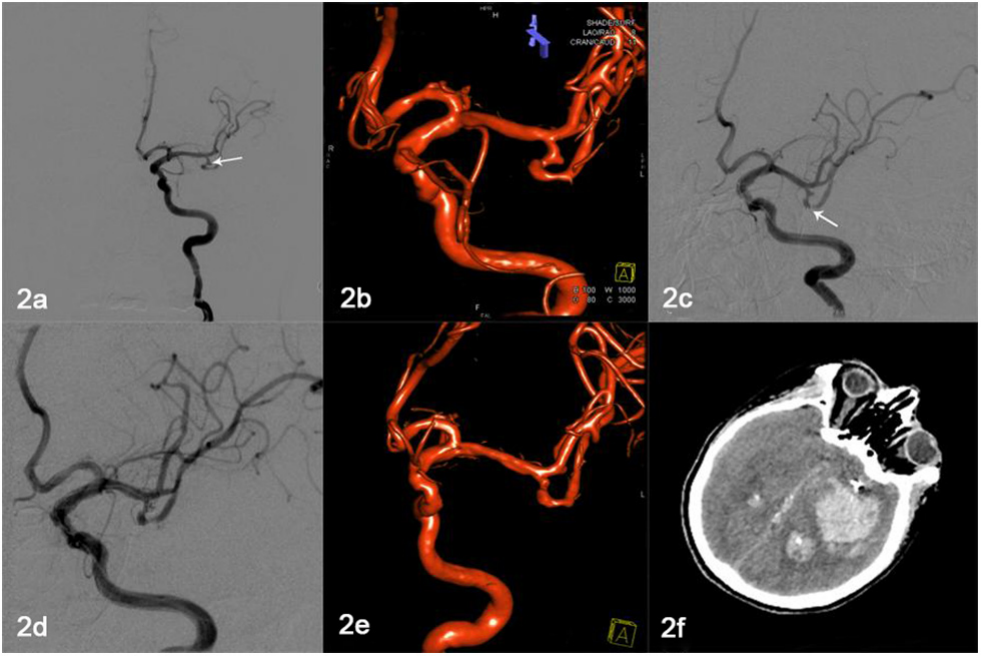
Hemorrhage after stenting in one patient. (a–b) Figure depicts a 65-year-old man with a left MCA ruptured wide-necked aneurysm (2.5 mm × 3.2 mm) (white arrow). (c–e) Solitaire AB stent (4 mm × 20 mm) (white arrow) assisting complete occlusion; there was parent artery patency. (f) CT revealing hemorrhage in the left MCA area 5 h after surgery.
